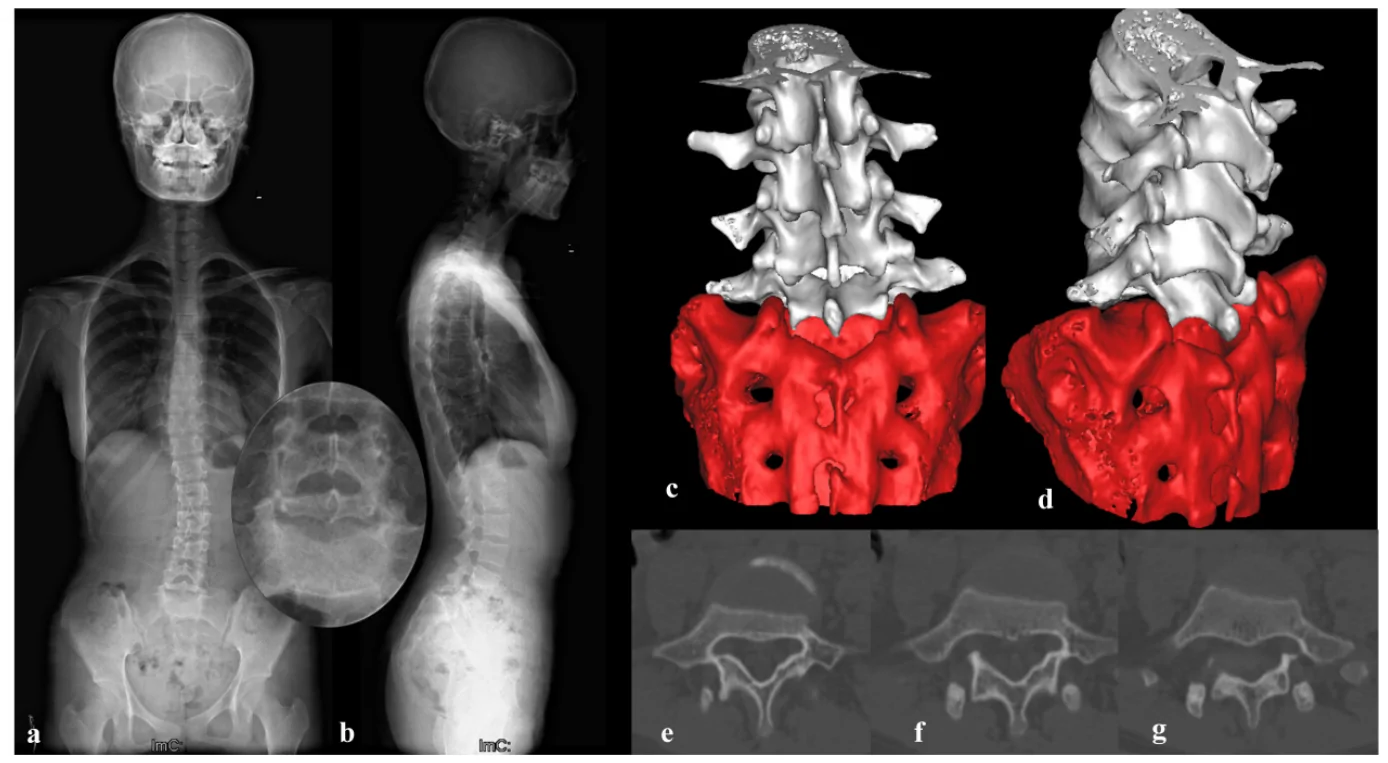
Preoperative radiographic and computed tomography (CT) findings. (a) Standing anteroposterior full-spine radiograph showing a mild thoracolumbar scoliotic curve. (b) Standing lateral radiograph showing Meyerding grade I anterolisthesis of L5 on S1 and mild rounding of the sacral endplate. (c,d) Posterior and oblique three-dimensional CT reconstructions showing preserved pars interarticularis morphology and anterior translation/subluxation of the L5 inferior facets relative to the S1 superior facets. (e–g) Consecutive axial CT images demonstrating nearly sagittal orientation of the L5-S1 facet joints.
